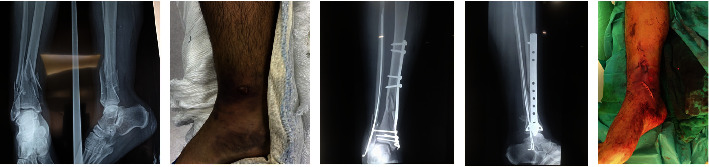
Pre- and postoperative clinical and radiographic images of a 33-year-old patient treated with MIPO.
